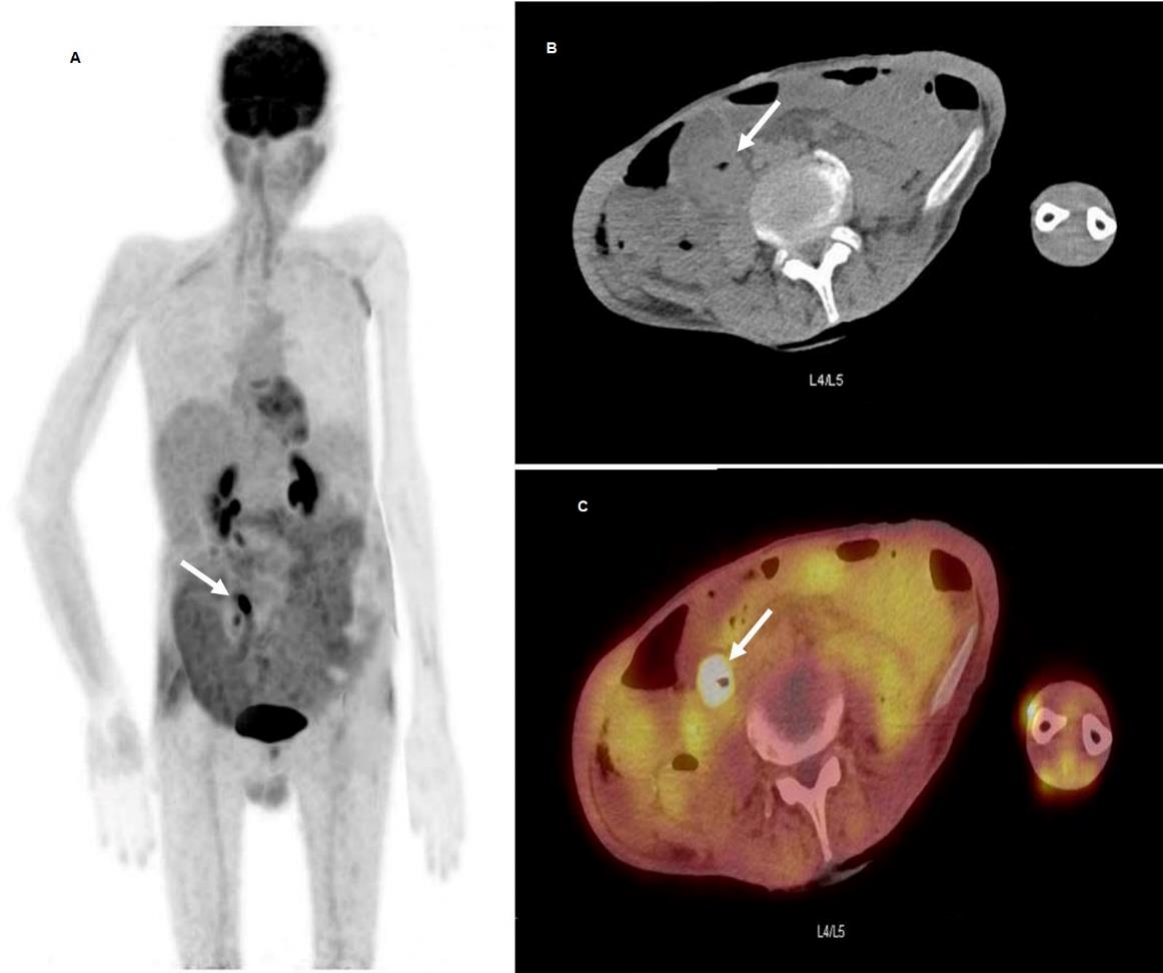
^18^F-FDG PET/CT scan performed on April of 2021 and showed single short segmental hypermetabolic process (arrows) within the ileum, manifestation of inflammatory bowel disease vs more serious pathologies such as Enteropathy-associated T cell lymphoma (EATL) or recurrent disease were suspected. Seen on the maximum intensity projection (MIP) image (A), as well as on axial CT (B) and on axial fused PET/CT (C) images at the level of L4/L5 vertebrae
